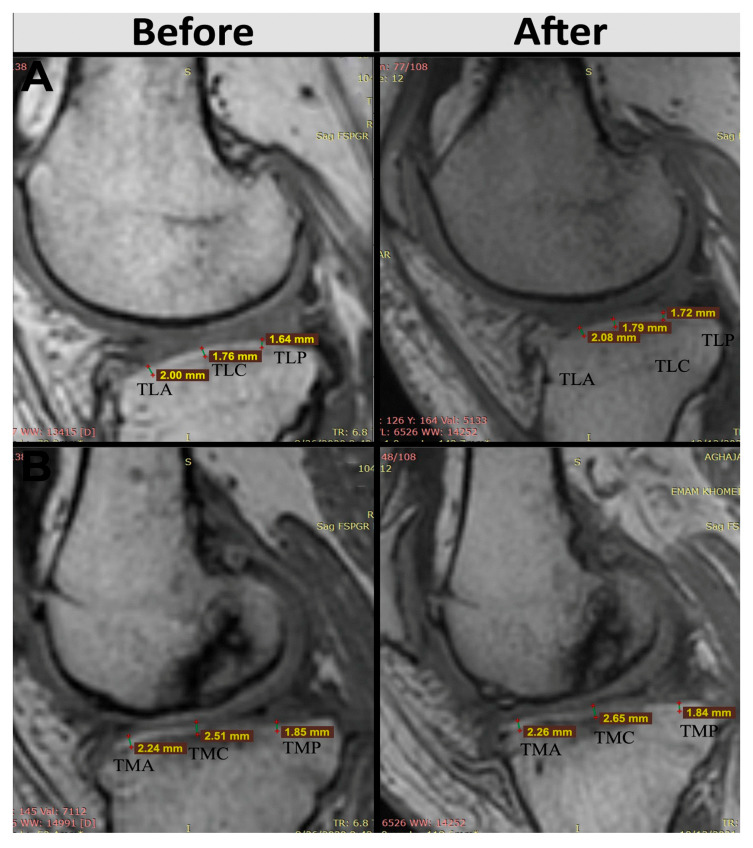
MRI assessment of the tibial condyle cartilage before and 48 weeks after AASCs injection. (A) The lateral view shows increased cartilage thickness in the anterior, posterior and central regions of the tibial condyle. (B) The medial view highlights an enhancement in cartilage thickness across the anterior, posterior and central areas of the tibial condyle. MRI: magnetic resonance imaging; TLA: tibia lateral anterior; TLC: tibia lateral central; TLP: tibia lateral posterior; TMA: tibia medial anterior; TMC: tibia medial central; TMP: tibia medial posterior; AASCs: adipose-derived mesenchymal stromal cells. (Reprinted without modifications from Sadri et al. [32]).
